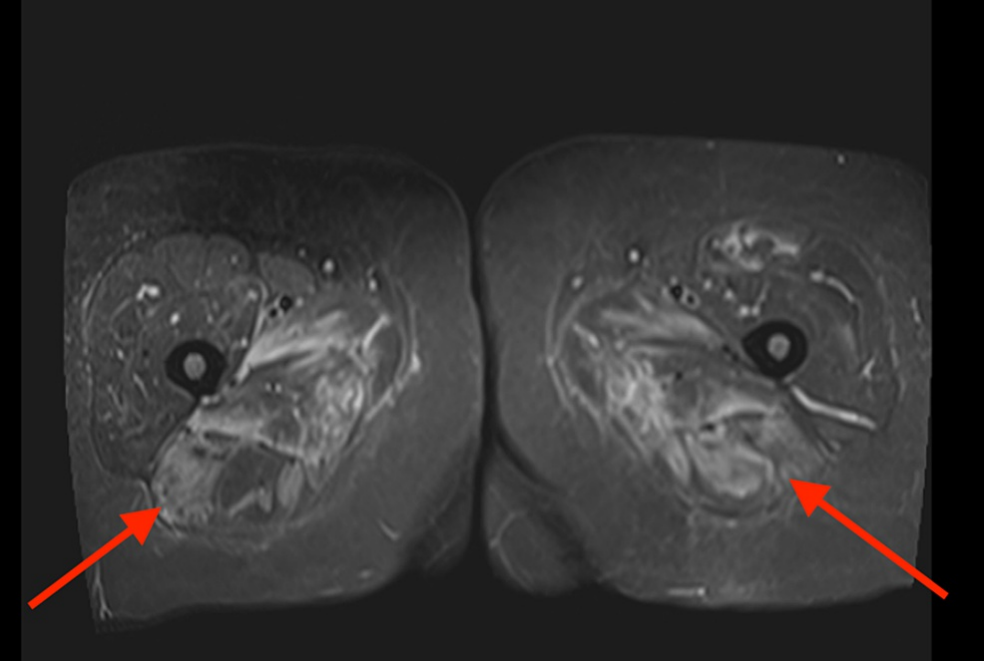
MRI Axial STIR sequence. Edema in bilateral posterior thigh muscles and surrounding fascia. STIR: Short tau inversion recovery.
